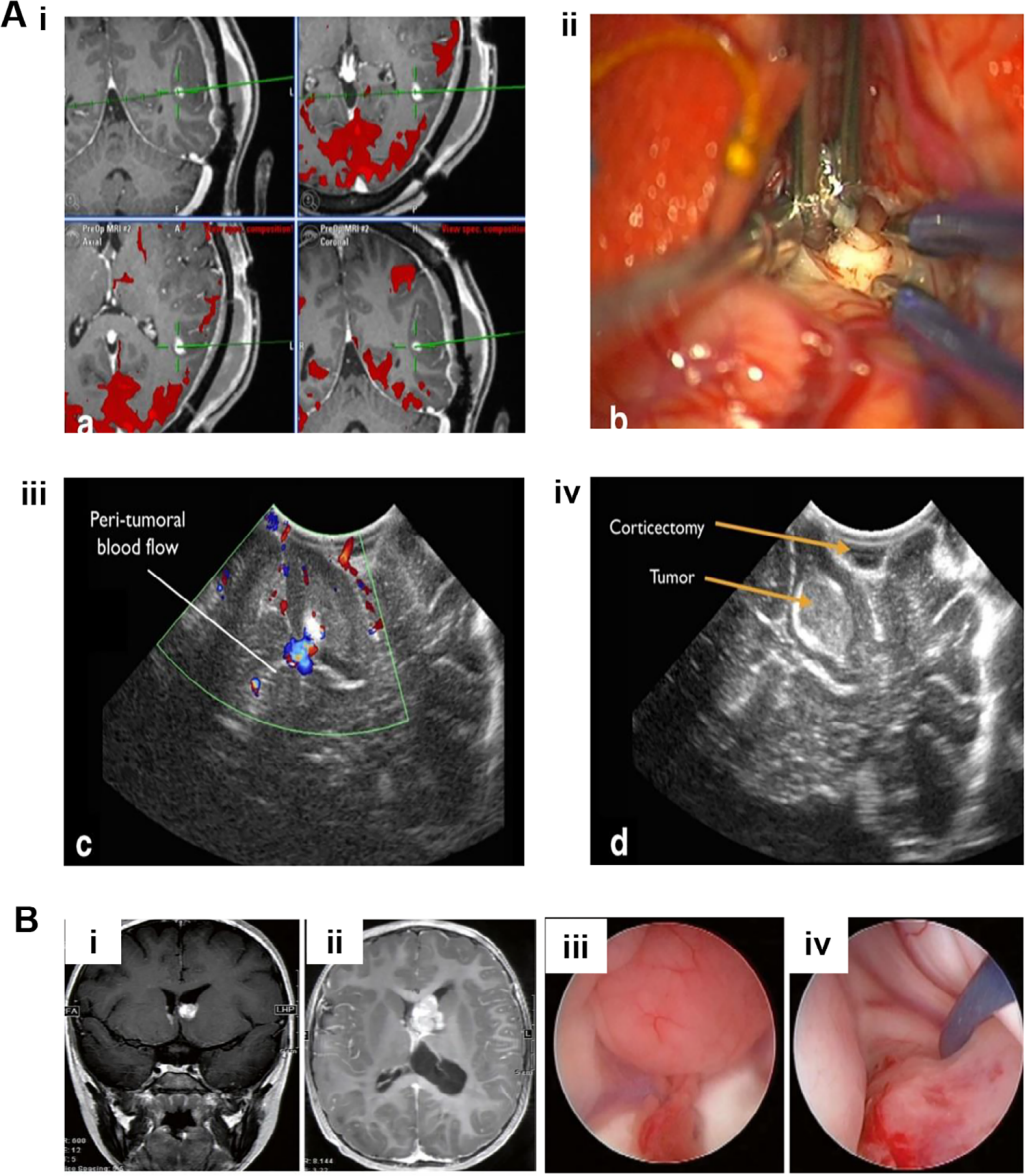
Surgical management for a brain tumor. (A) Craniotomy: A subcortical lesion in the left angular gyrus (i). Real‐time intraoperative ultrasound was used to precisely locate the lesion (ii) and surrounding blood vessels (iii). Minimal disruption to the surrounding cortex during lesion removal (iv). Reproduced with permission.^[^
